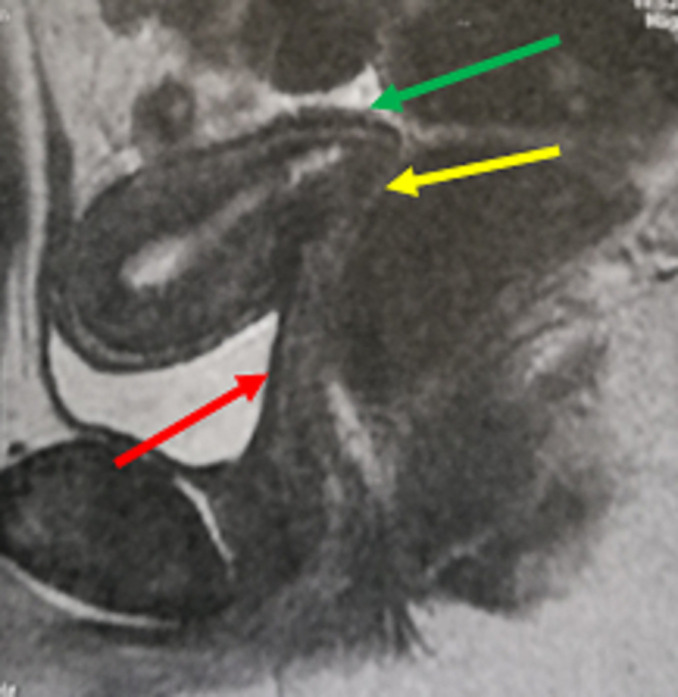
magnetic resonance imaging scan showing the vagina (in red), the isthmus (in green) and the absence of cervix (in yellow)
